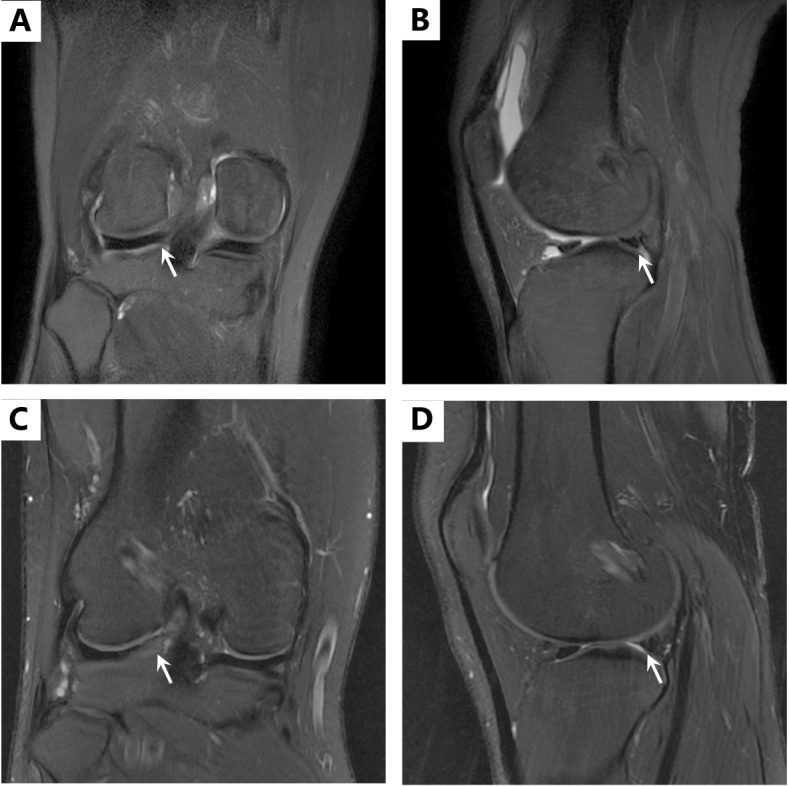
Healing status on magnetic resonance image 2 years after LMPR repair, white arrows showed the posterior root of lateral meniscus. A, B Complete healing: an identifiable meniscus on both sagittal and coronal scans. C, D Partial healing: an identifiable meniscus was showed but high signal could be detected among the normal dark meniscal signal on both sagittal and coronal scans (*LMPR* lateral meniscus posterior root)
